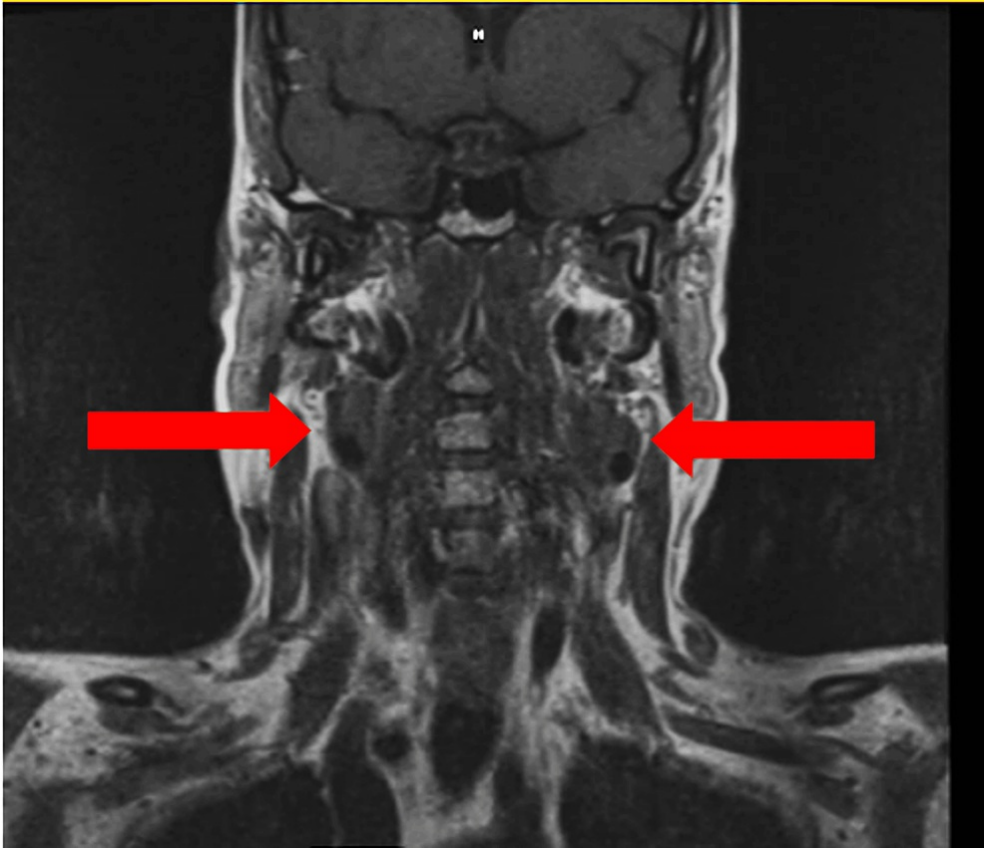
Coronal T1-weighted MRI showing bilateral carotid body tumors (red arrows) at the level of the carotid bifurcation
